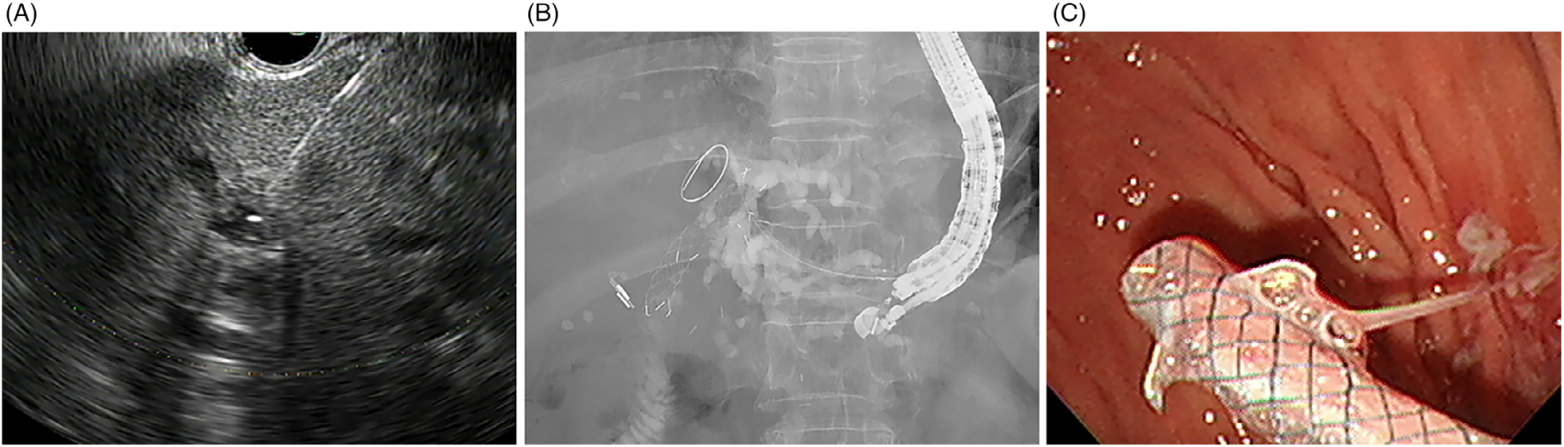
Endoscopic ultrasound‐guided hepaticogastrostomy. (A) Endoscopic ultrasound image showing the dilated left intrahepatic duct punctured by a 19G needle. (B) The fully covered metal stent (FCMS) was advanced through the guidewire and deployed using the intrascope release technique. (C) Duodenoscopic view showing the gastric ends of FCMS after successful deployment.
